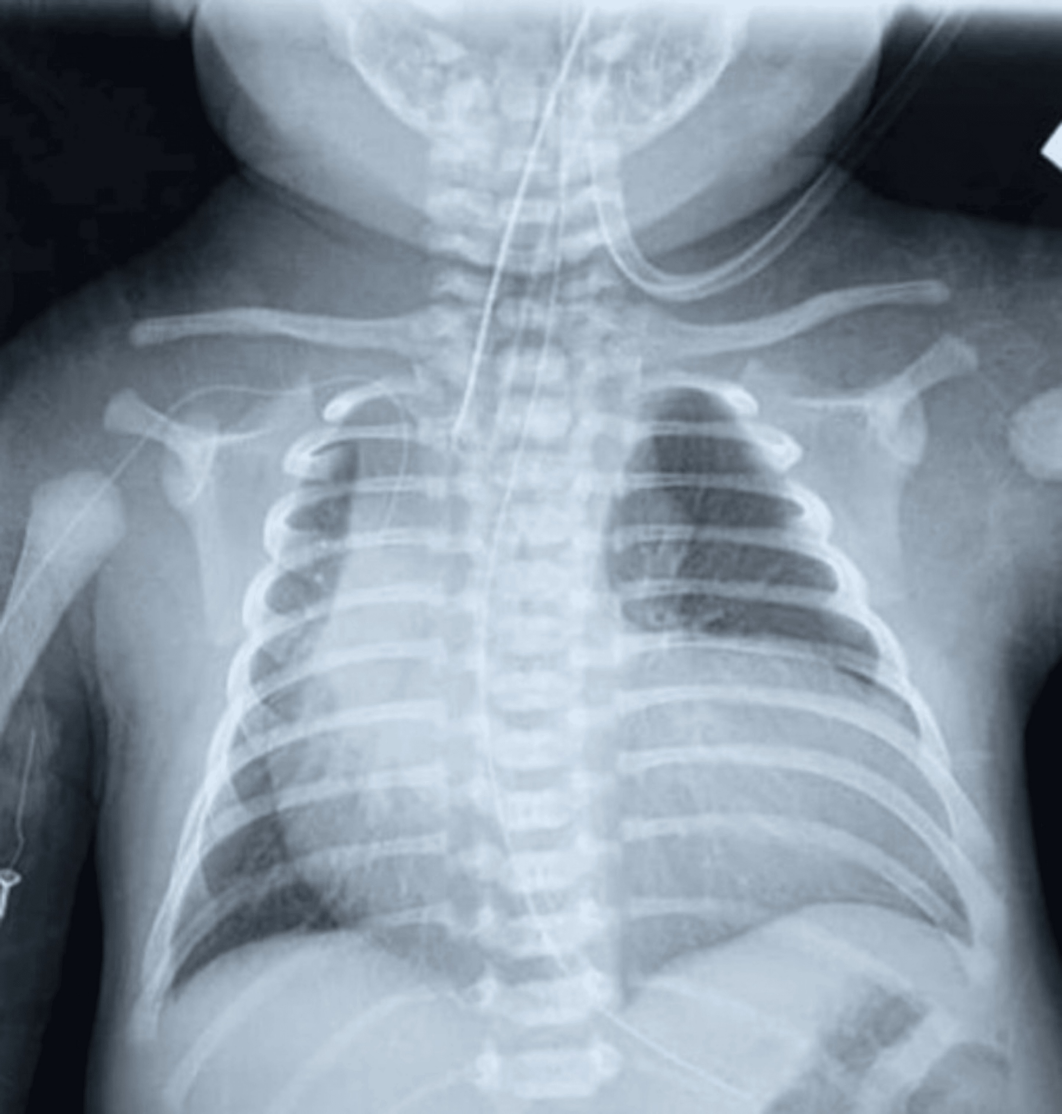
Radiographic evaluation showed marked cardiomegaly and a boot-shaped cardiac configuration. Furthermore, there was evidence of increased pulmonary vascular markings (pulmonary plethora), suggesting pulmonary overcirculation.
